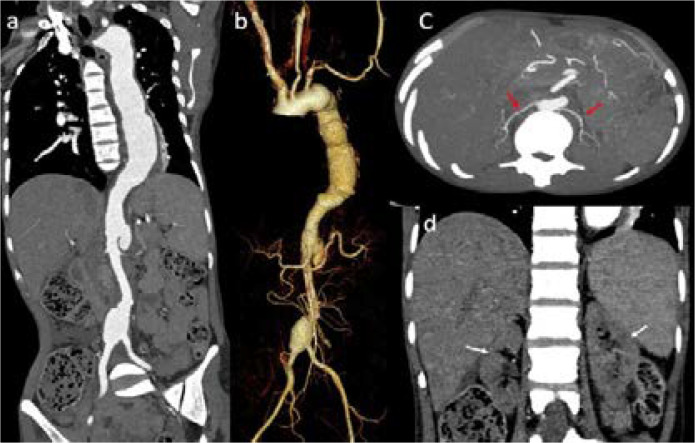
Computed tomography aortic angiogram Legend: (a) Coronal image shows diffuse circumferential mural thickening of descending thoracic and abdominal aorta. (b) 3D-Volume rendered (VR) image depicts multifocal ectasia and aneurysmal dilatation of descending aorta with few saccular outpouchings. (c) Axial maximum intensity projection (MIP) image shows bilateral thin calibre renal arteries (red arrows). (d) Coronal image showing bilateral small kidneys (white arrows).
